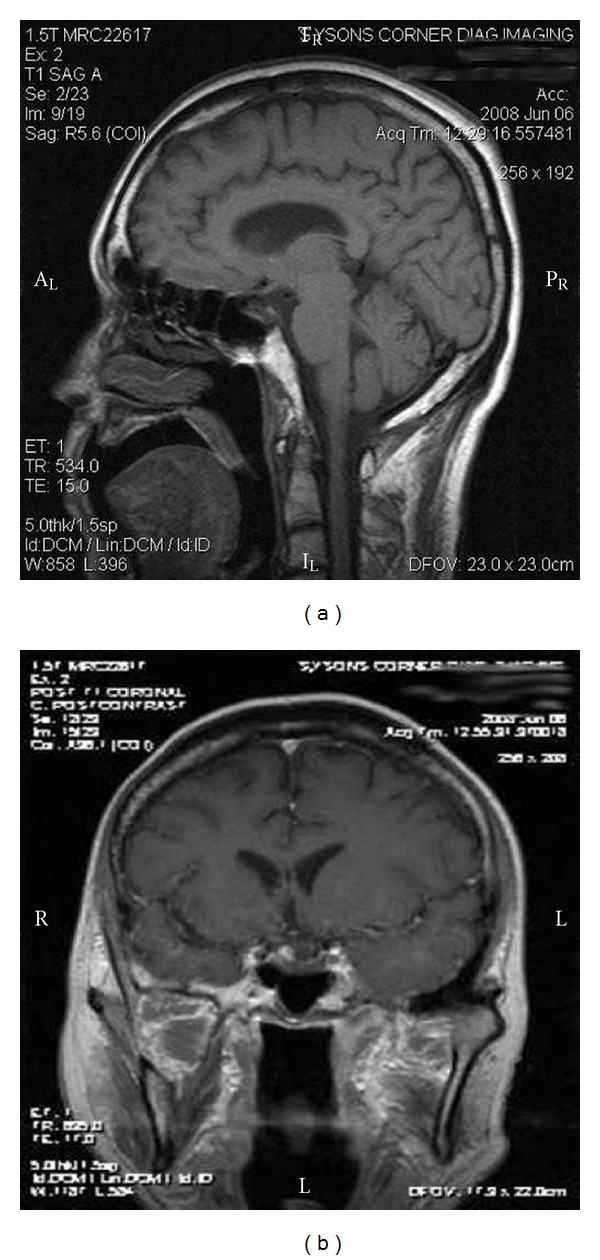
Sagittal and coronal postcontract MRI in early June revealing an empty sella without evidence of pituitary mass or cystic lesion.
